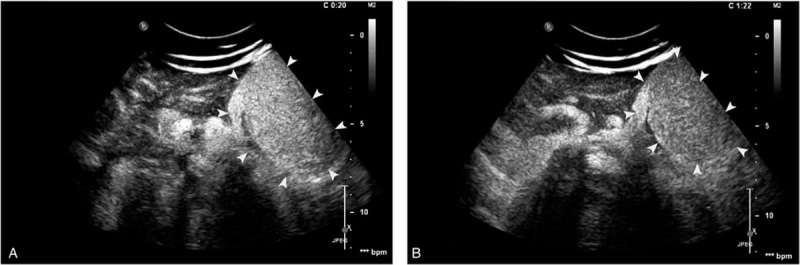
A. contrast-enhanced ultrasound: a remarkable quick enhancement of the mass (white arrow heads) compared to the liver in the arterial phase. B. contrast-enhanced ultrasound: the enhancement of the mass (white arrow heads) was almost isoechoic to the liver parenchyma during the portal and late phase.
